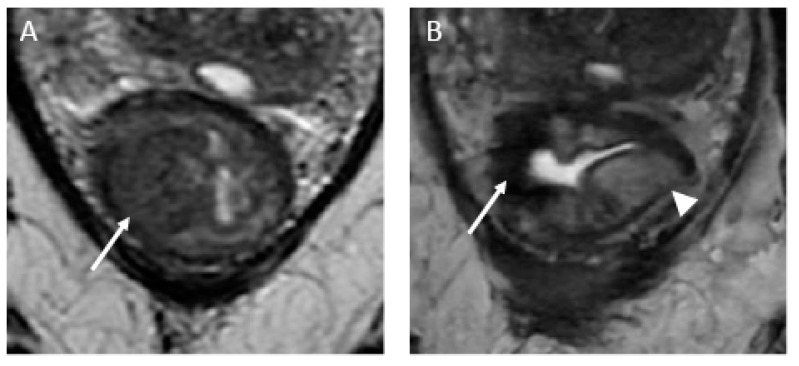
Submucosal edema mimicking residual tumor in the uninvolved rectal wall in a 47-year-old man with rectal adenocarcinoma. (A) Baseline axial T2-weighted image shows a semicircumferential rectal tumor extending between the 7 o’clock and 12 o’clock positions (arrow). (B) Axial T2-weighted image after the completion of neoadjuvant chemoradiotherapy shows a complete response appearance, characterized by a crescent-shaped scar within the mucosal/submucosal layers (arrow). Note the high signal intensity at the uninvolved rectal wall (arrowhead), representing submucosal edema. Response assessment should not be based on the appearance of uninvolved parts of the rectum.
